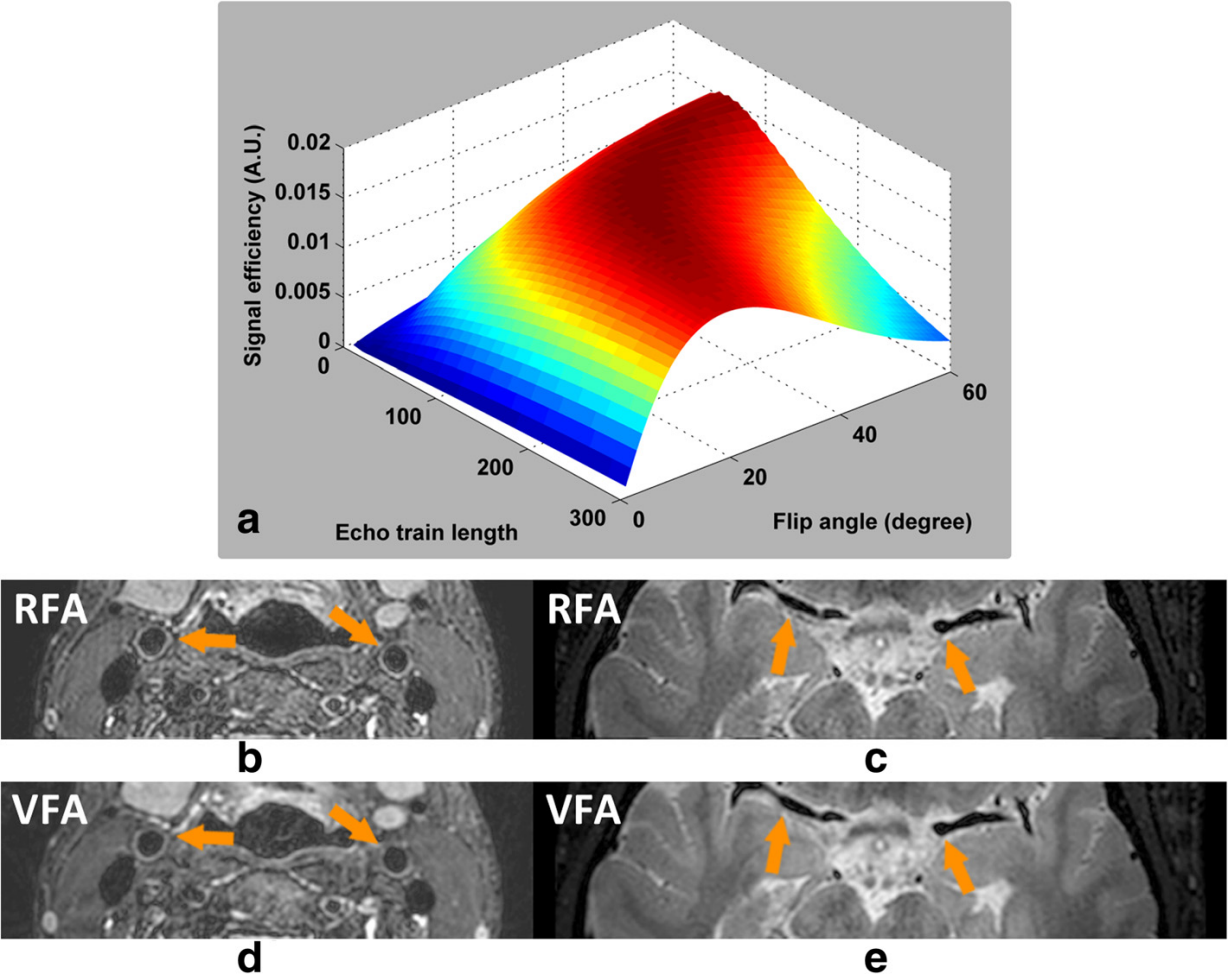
Optimization of T2-weighted VISTA echo train. Panel (a) shows the optimization of RFA scheme on echo train length and refocusing flip angle. The optimized RFA scheme (b, c) can further improve the sharpness of vessel wall boundary (marked in yellow arrows) but cause SNR loss compared to VFA scheme (d, e)
